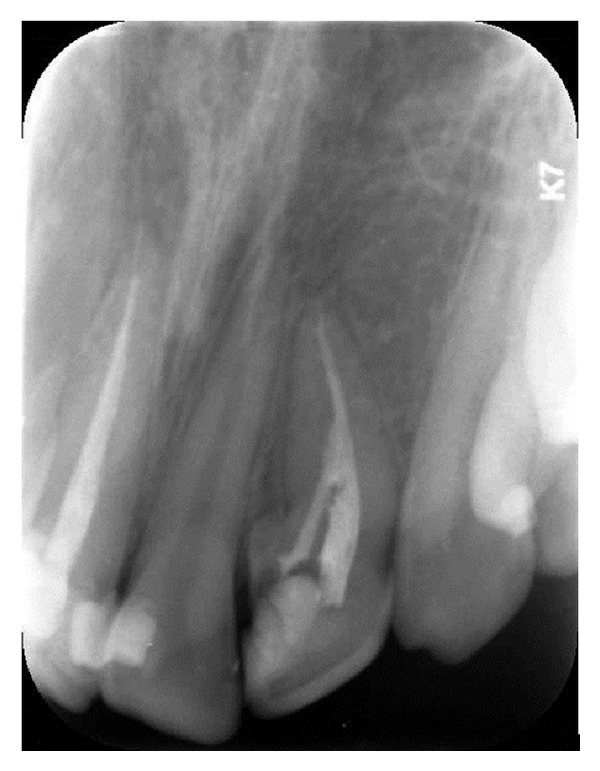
Recall radiograph after 6 months.
